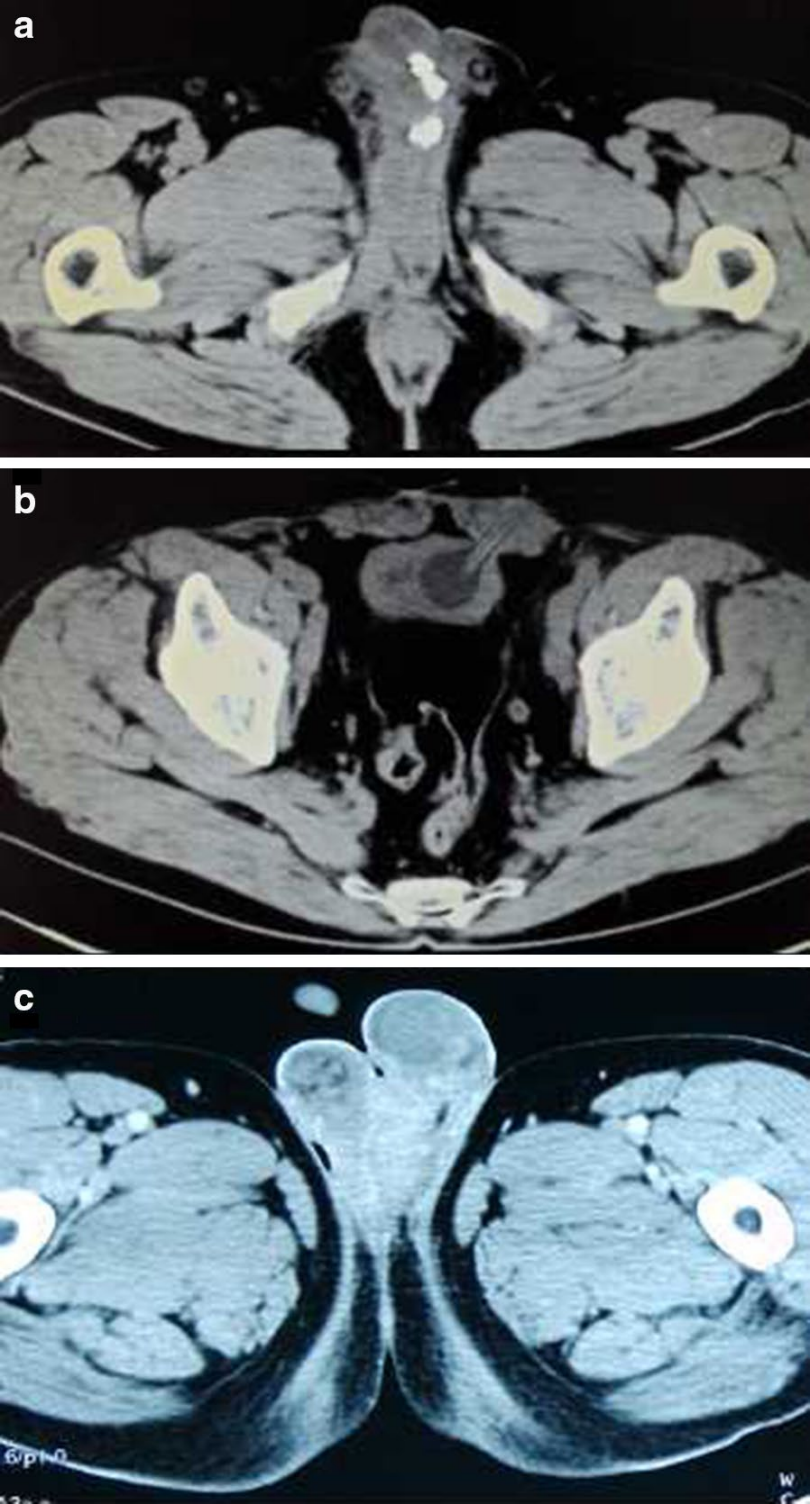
a Computed tomography scan of the soft tissue in the penis showing multiple calculi in the distal urethra. b Computed tomography scan of the pelvis showing a thickened urinary bladder and Foley’ s catheter through the abdominal wall. c Computed tomography scan of the scrotum showing a purulent infection and its spread in the perineal fascia space
